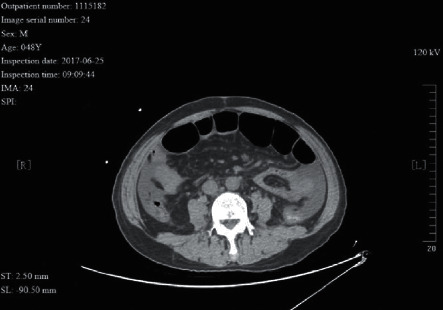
A 48 year-old male patient with adhesive intestinal obstruction. He had received “radical resection of rectal cancer”. The CT image shows signs of ascites. Partial bowel wall ischemia of the small intestine proximal to the obstruction was seen during surgery.
